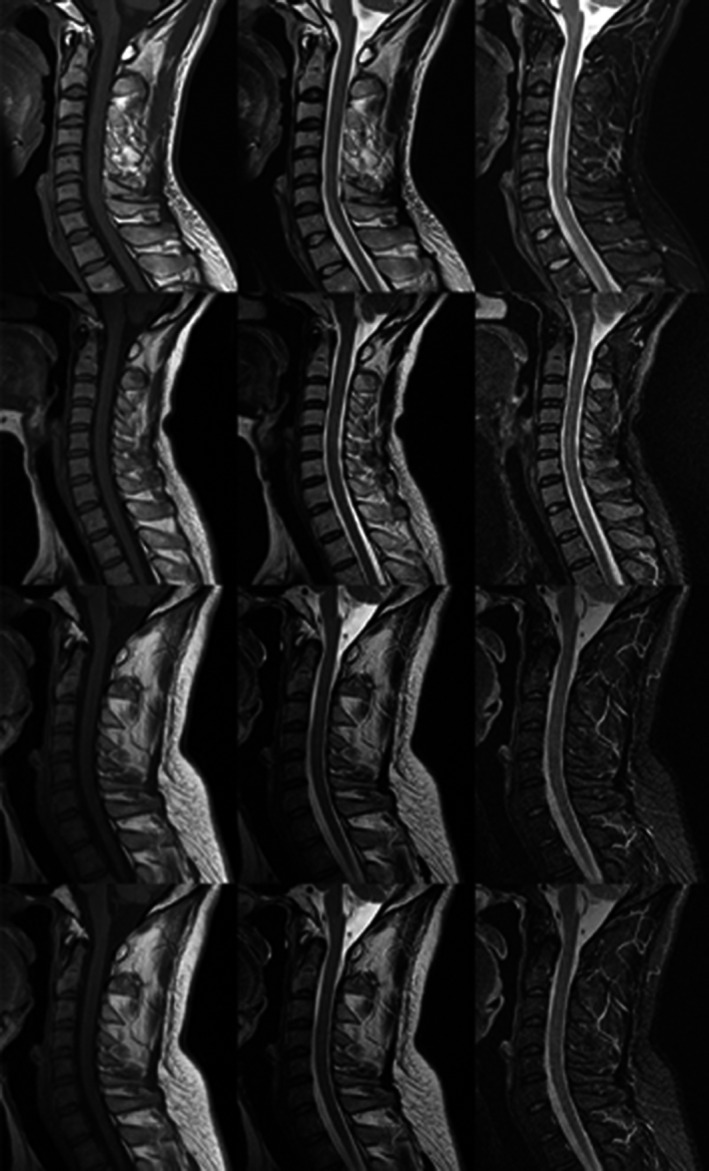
Comparison between normal magnetic resonance imaging (MRI) and abnormal MRIs.
